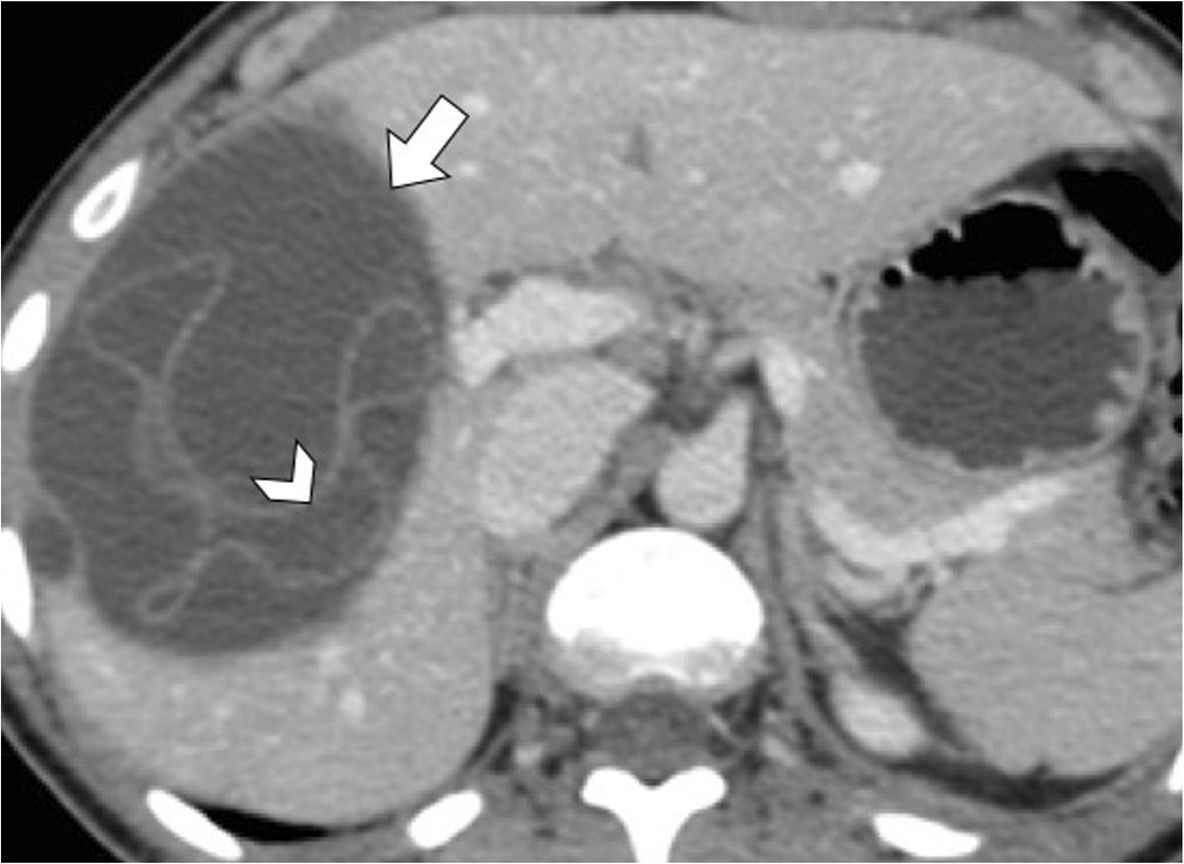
A 50-year-old woman with contained rupture hydatid cyst. Axial contrast-enhanced CT on portal venous phase shows hepatic hydatid cyst (arrow) with hyperattenuating serpentine linear membrane within the cyst (arrowhead)
